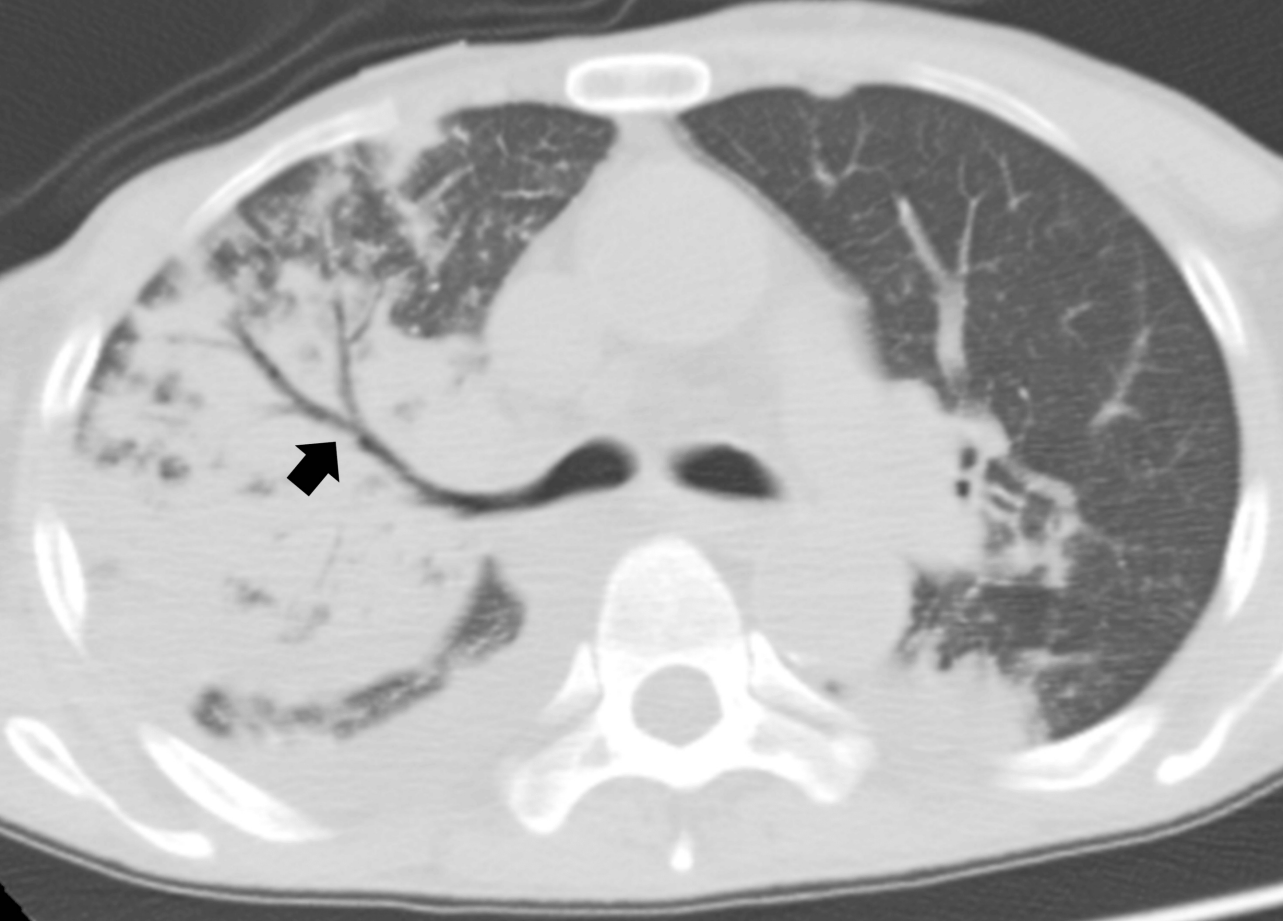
Chest CT scan Chest CT revealed an extensive infiltrative shadow with an air bronchogram (arrow) in the right lung.
